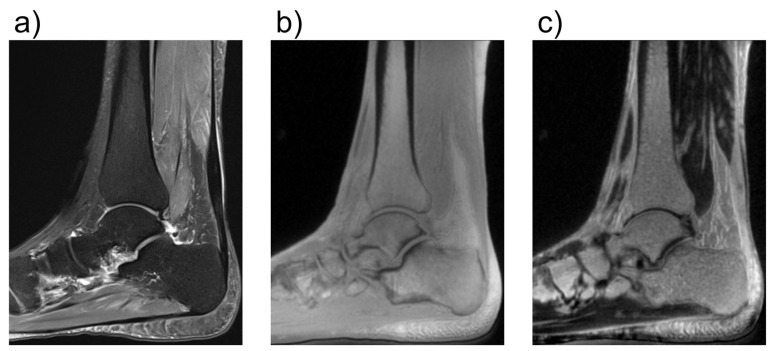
Comparison of conventional and ultrashort echo time (UTE) MRI images of the foot in the sagittal plane. In (a), a fat-saturated proton-density weighted image is shown with an echo time (TE) of 42 ms. In (b), a UTE image with a TE of 0.05 ms is shown. In (c), a subtraction image is shown, in which the signal of an image with TE = 4 ms is subtracted from the image in (b). While in (b), all structures have very high signal because of the short TE, in (c) only the images with very short T2*-times are shown as bright. With regard to cartilage, this enables better assessment of the health of the deepest calcified cartilage layers. The UTE images were acquired using a density-adapted 3D radial (DA-3D-RAD) imaging sequence.
